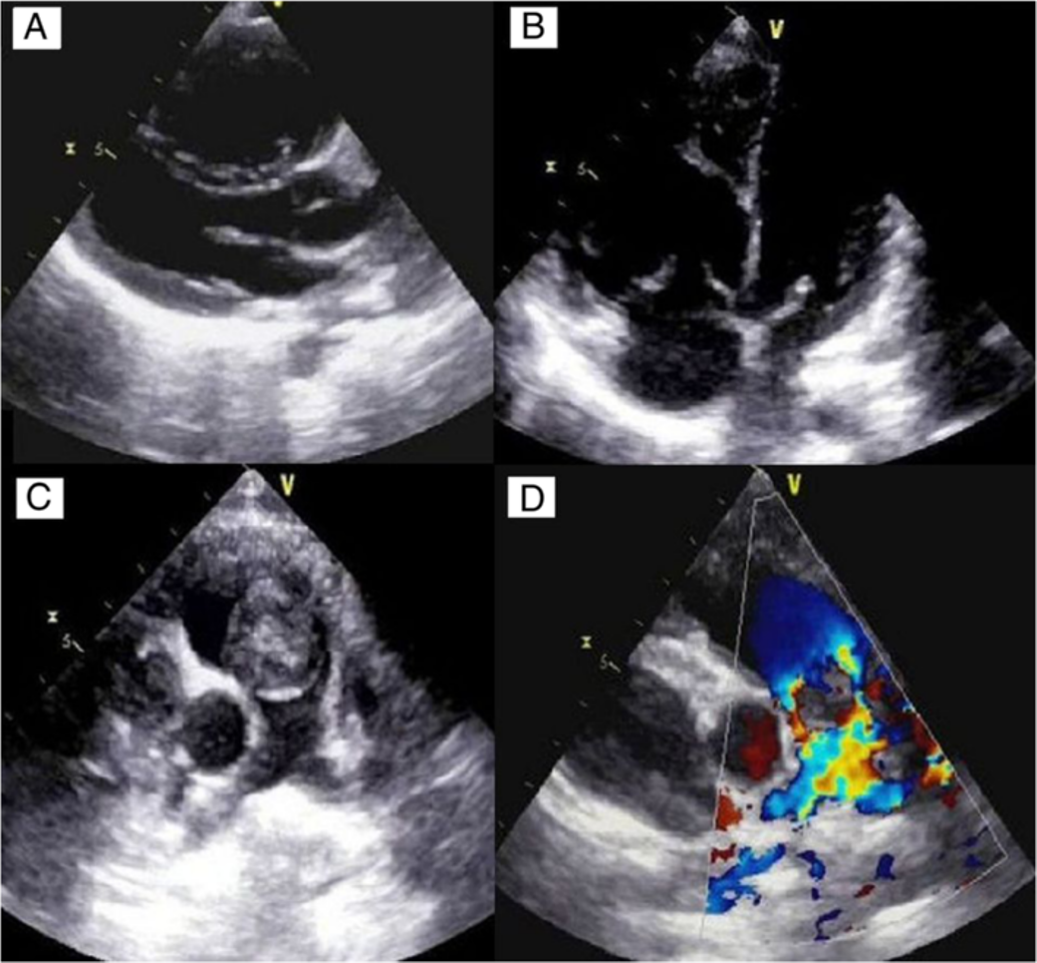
Transthoracic echocardiography. Parasternal long-axis view (panel A) and apical four chambers view (panel B) showing right ventricular enlargement. Parasternal short-axis view at the level of the aortic valve reveals the right ventricular outflow tract tumor (panel C). The obstructive character of this tumor is demonstrated by color Doppler in the same view (Panel D).
